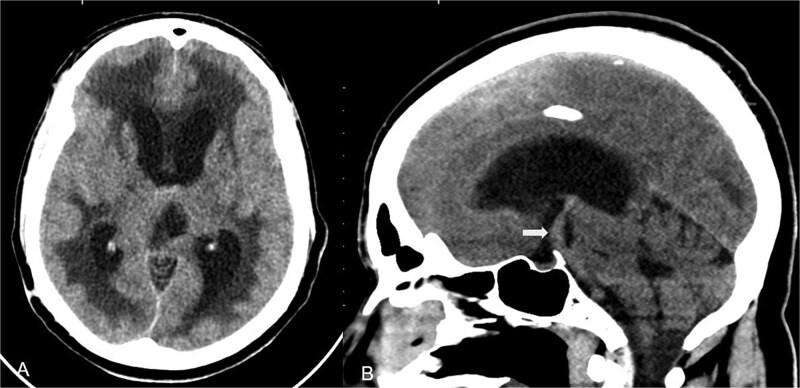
Non-contrast CT of the brain at presentation. (A) Axial CT image at the level of the lateral ventricles demonstrating ventriculomegaly with periventricular hypodensity consistent with transependymal cerebrospinal fluid (CSF) permeation. (B) Mid-sagittal CT reconstruction showing an elongated and ectatic basilar artery indenting the floor of the third ventricle.
